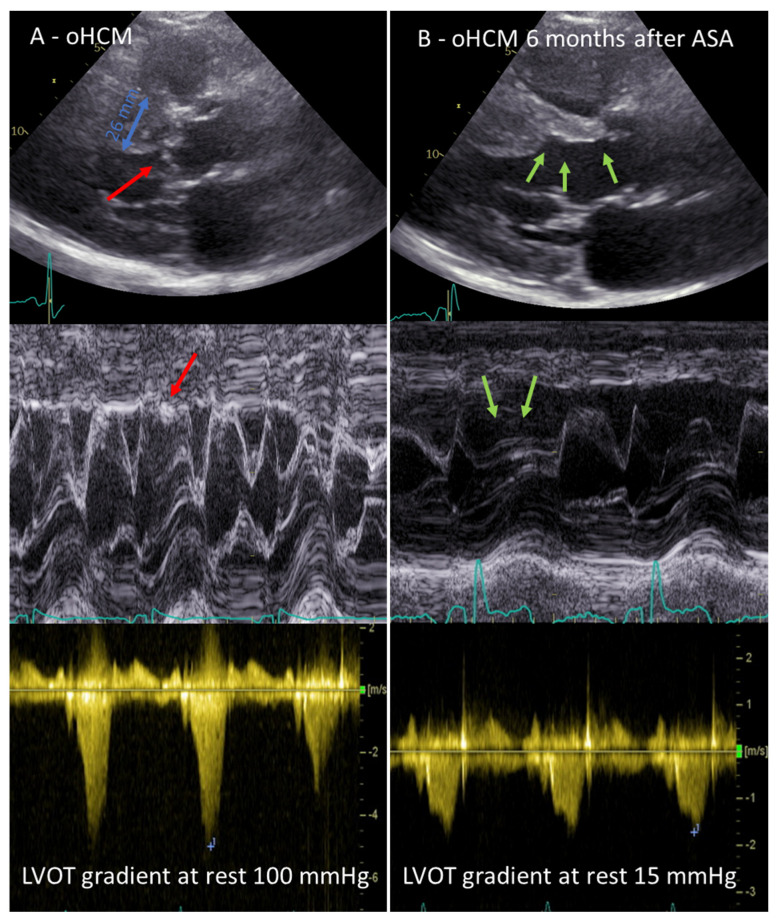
(A) Echocardiographic features of obstructive hypertrophic cardiomyopathy (oHCM; septal bulge with maximum diameter of 26 mm; red arrows indicate systolic anterior movement phenomenon of the mitral valve with contact to the septal bulge, left ventricular outflow tract [LVOT] gradient at rest of 100 mmHg); (B) Echocardiographic result 6 months after alcohol septal ablation (ASA), green arrows indicate the thinning of the basal septum, resolution of SAM and LVOT gradient at rest of 15 mmHg.
